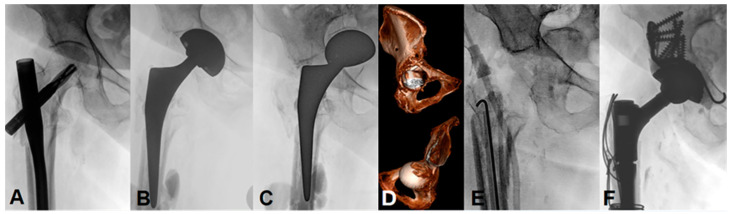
Anteroposterior radiographs and computed tomography (CT) images of an 80-year-old woman with (A) a symptomatic non-union of a pertrochanteric fracture on the right side, ten months after the osteosynthesis with a femoral nail. (B) Postoperative radiographic view two days after a THA with an interruption of the ilioischial and iliopectineal lines. (C) Atraumatic acetabular fracture after partial weight-bearing four weeks postoperative with a complete intrapelvic protrusion of the acetabular component. (D) A corresponding CT scan was acquired for evaluation of the fracture pattern (AAOS IIA) and the preoperative planning. (E) Two-stage revision with the removal of the femoral and acetabular components and the placement of an antibiotic-cement spacer due to the concomitant diagnosis of chronic prosthetic joint infection. (F) Reconstruction of the acetabulum using a dual mobility acetabular component, a structural and morselized bone graft, and a Ganz reinforcement ring, six months after the first stage of the revision.
